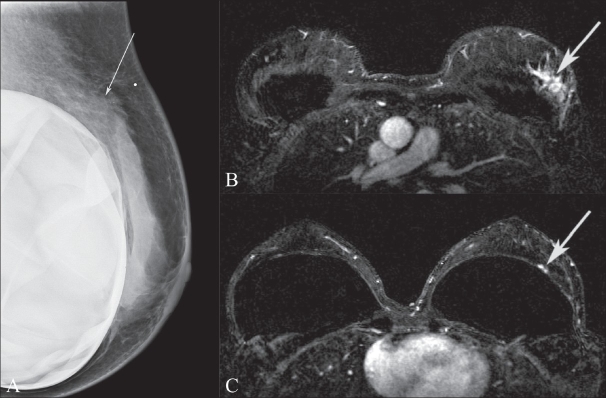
A 45-year-old female with a new palpable abnormality that was proven to be invasive ductal carcinoma. A mediolateral oblique mammographic projection of the left breast (A) with a BB in place at the site of the palpable abnormality demonstrates increased density (arrow) with associated calcifications. Preoperative DCE-MRI was performed (B,C), which revealed the breast carcinoma (arrow in B) as well as an additional focus of disease at the 3 o'clock position (arrow in C) which was not seen on mammography but was identified on second-look USG
